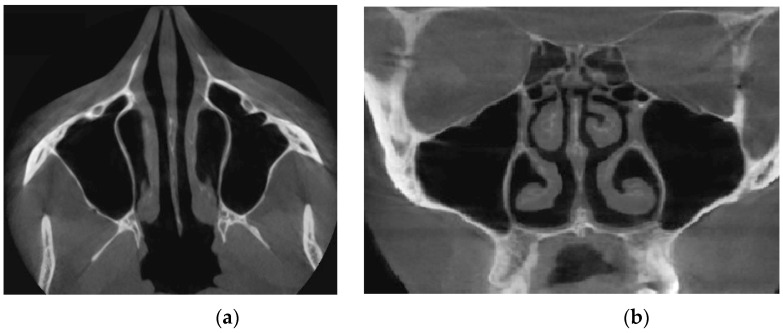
Computed tomography of the patient at the conditional norm: (a) axial tomographic section; (b) multiplanar reconstruction in frontal projection.
